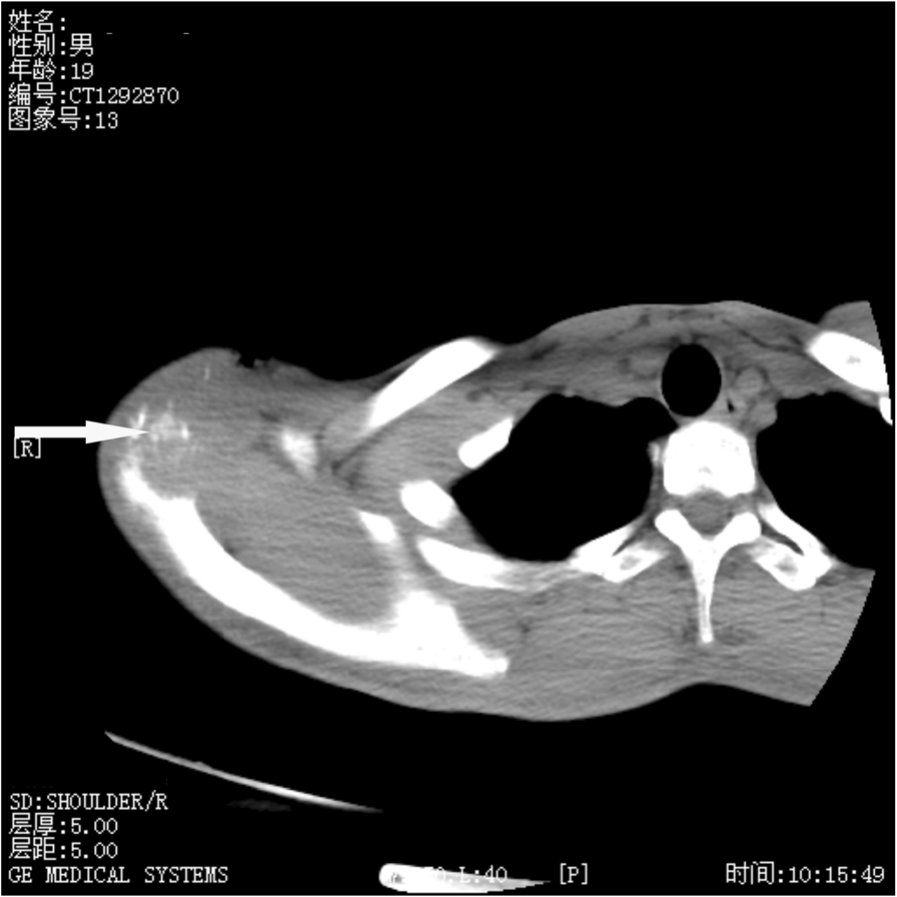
CT scan of right acromioclavicular joint showing erosions at the acromion process of scapula
